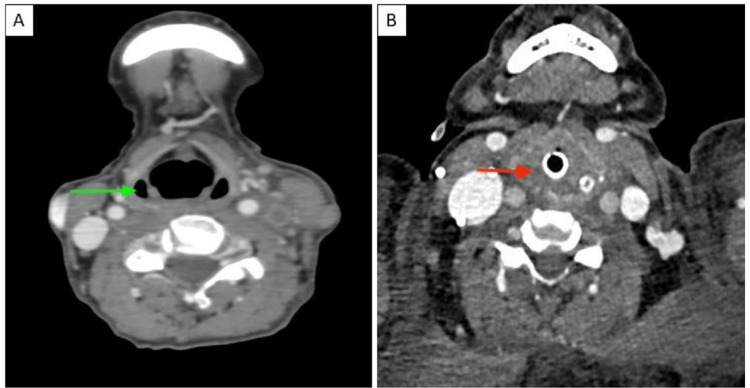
Axial CECT image of a normal patient (A) compared to our patient (B) Image A shows an axial CECT image of the neck showing normal pyriform sinuses (green arrow). In image B, the axial CECT image of the neck of the patient shows effaced pyriform sinuses due to edema in the hypopharynx/larynx (red arrow). The endotracheal tube is in situ. CECT: Contrast-enhanced computed tomography.
